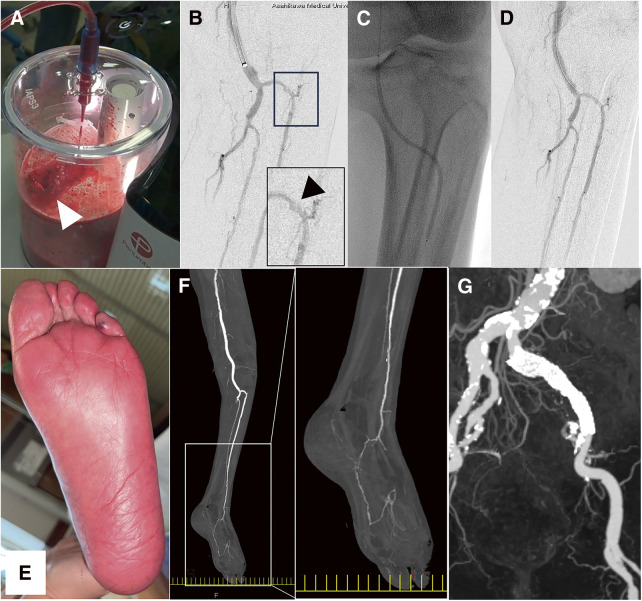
Fig. 3 The thrombus was effectively aspirated and captured in the engine (arrowhead, A). Posttreatment angiography of the CIAA revealed focal stenosis at the proximal ATA (indicated by the arrowhead, B). The arterial lesion was addressed with balloon angioplasty (C and D). His left leg was successfully salvaged (E), and postoperative CTA revealed well-revascularized arteries in the lower extremity (F) and left CIAA was covered with the stentgraft to prevent further embolization (G). CIAA: common iliac artery aneurysm; ATA: anterior tibial artery
